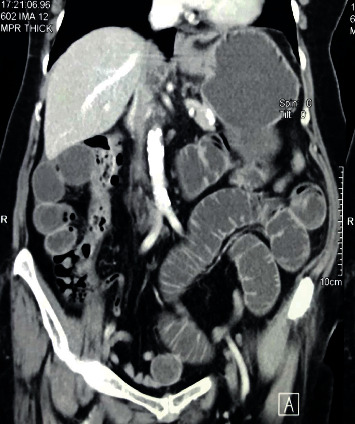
CT scan of the abdomen showing intestinal obstruction caused by Meckel's diverticulum.
